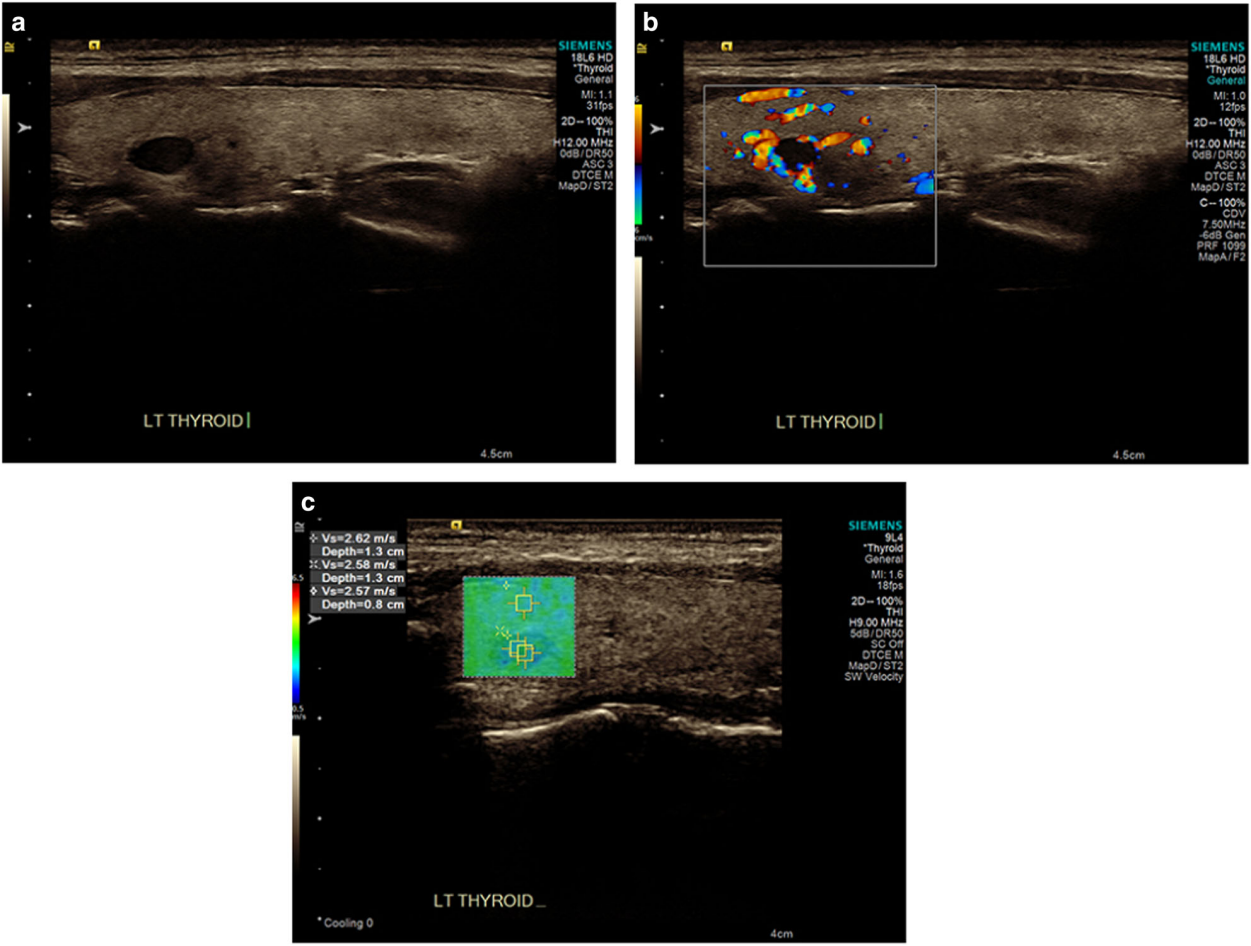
a An example of a B-mode image of a hypoechoic thyroid nodule in the left thyroid lobe measuring 7.2 × 4.6 × 4.4 mm. b Shows Color Doppler vascularity and c shows SWV measurements. Initial FNAB pathology was read as Atypia of Undetermined Significance (BC III); repeat FNAB confirmed this diagnosis and showed GEC-suspicious results. Surgical pathology showed a follicular adenoma
